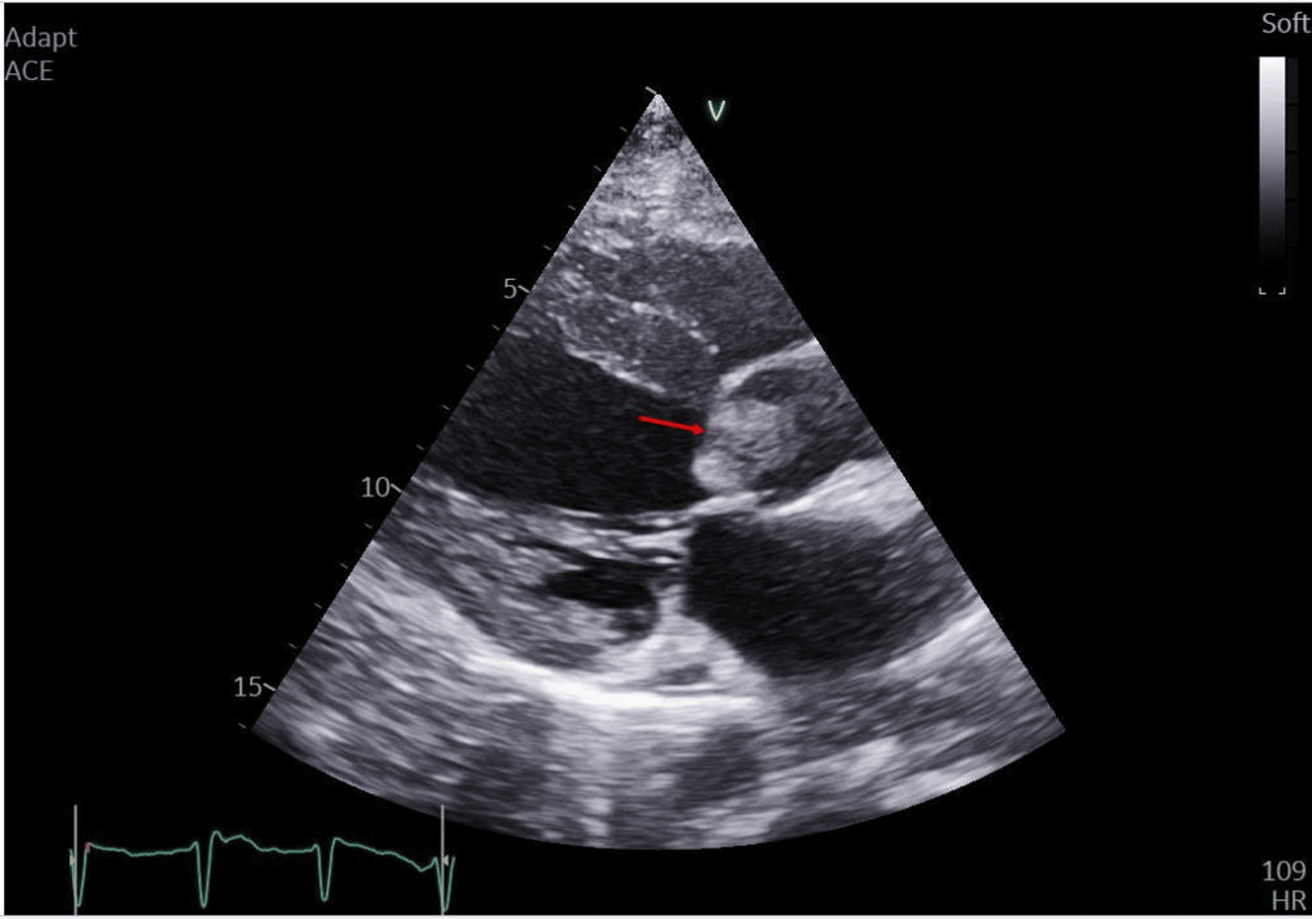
Transthoracic echocardiography (parasternal long axis view) showing the aortic valve mass measuring 2.3 x 1.4 cm (red arrow).
